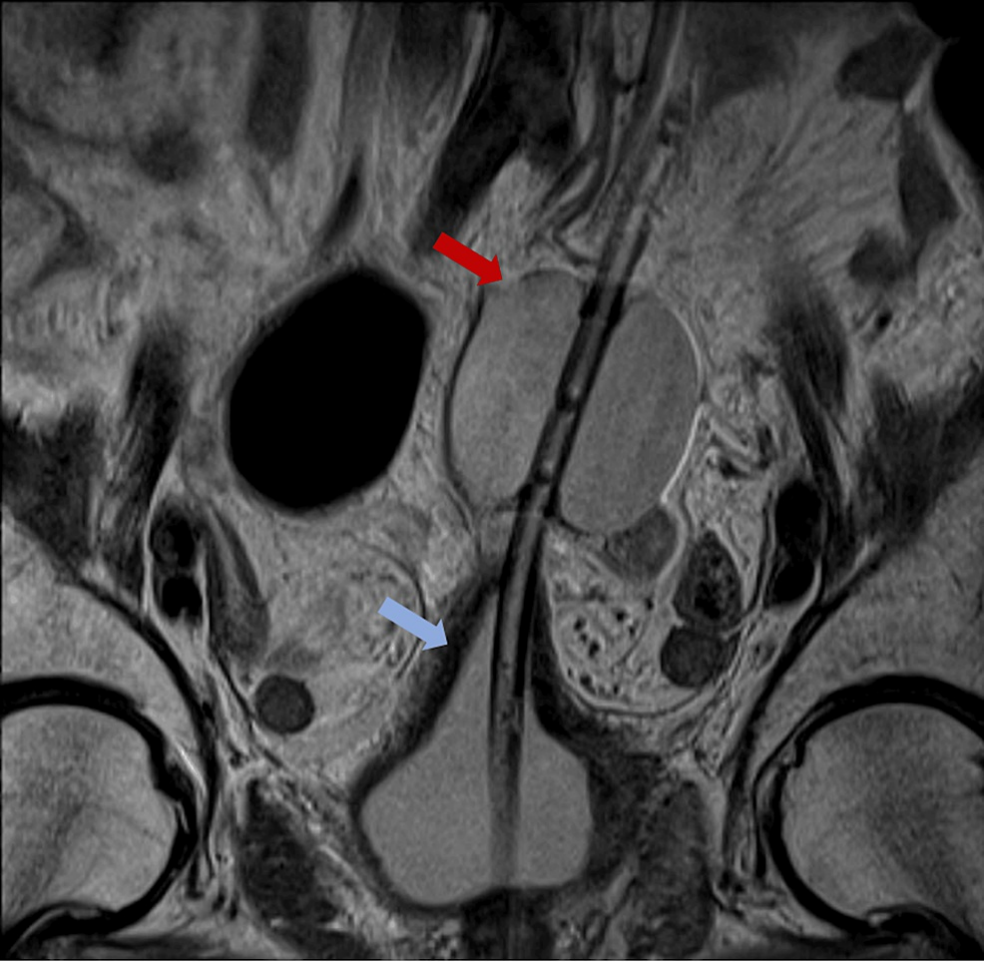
Coronal view in MRI of the bladder perforation. The red arrow points to the catheter balloon; the blue arrow points to the deformed bladder.
